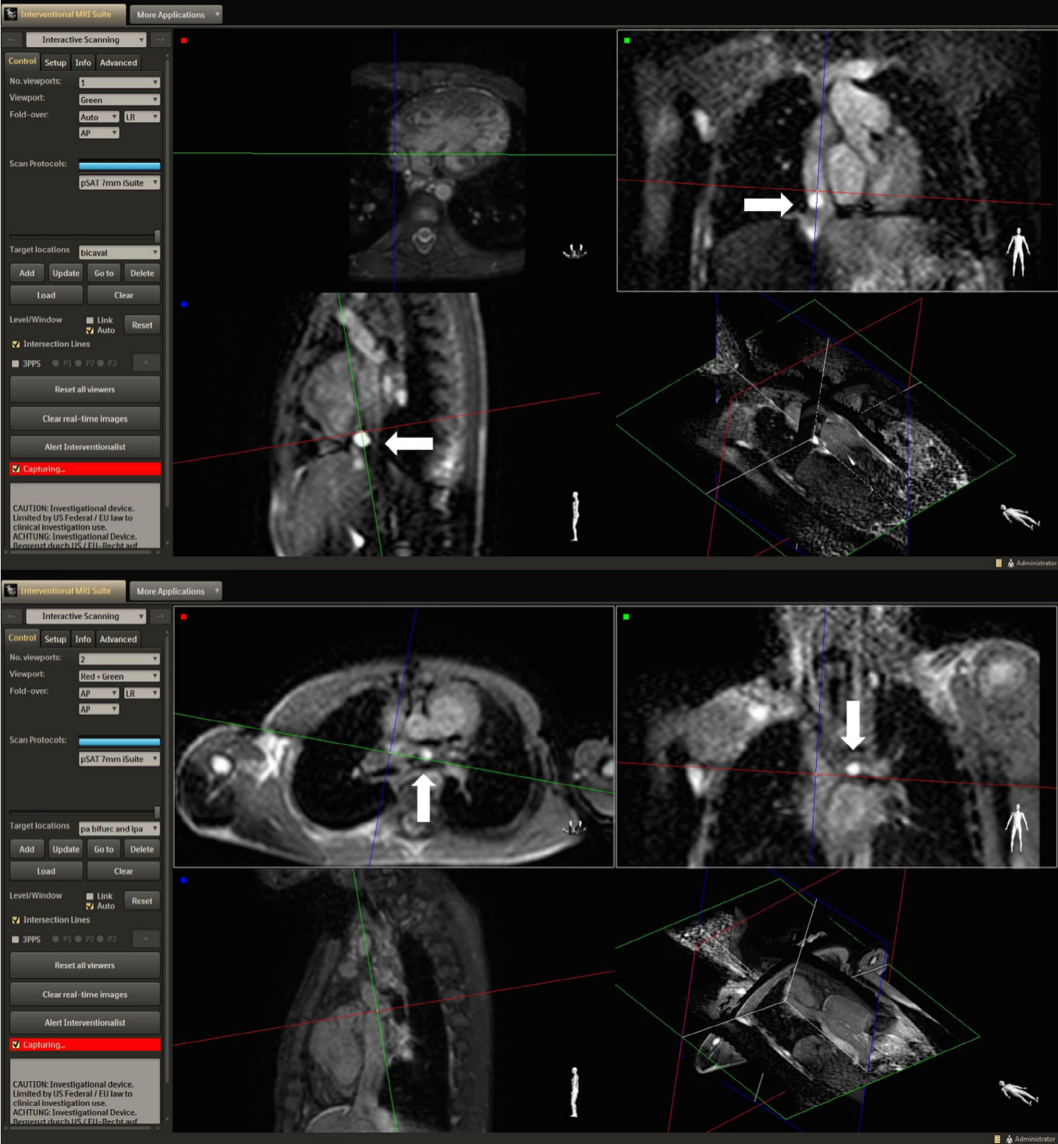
MRI‐guided catheterization of a patient with HLHS using the iSuite platform. The balloon‐tip is seen navigating the IVC (top image) and the LPA (bottom image) in a multiplanar viewer.
